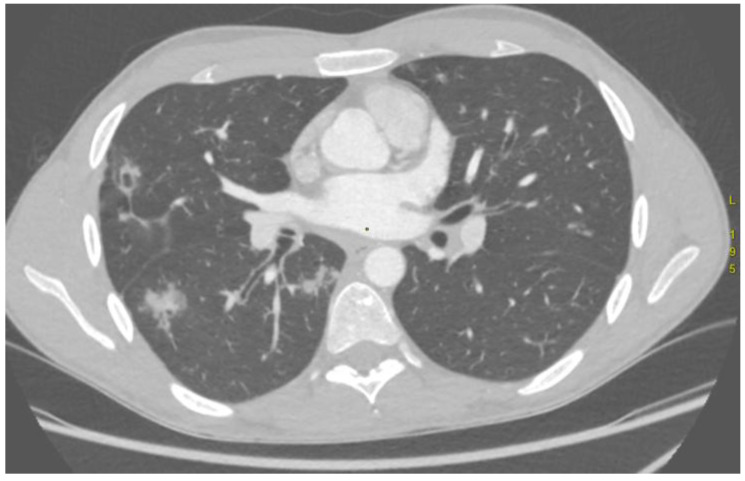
Computed tomography angiography demonstrates intrabronchial haemorrhage in the right lung, seen as hyperdense regions.
